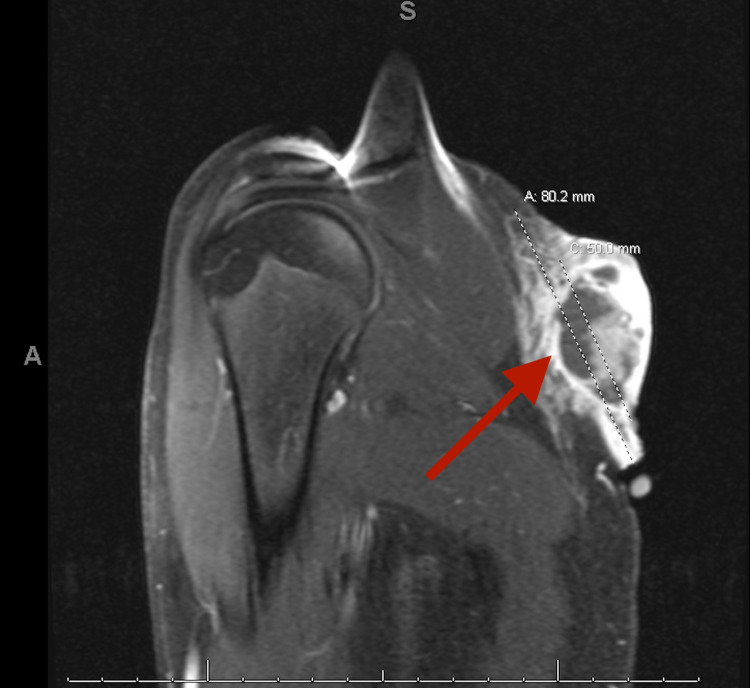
MRI of the mass Complex heterogeneously enhancing abnormality within the posterior upper left chest soft tissues with a multilobulated exophytic mass that protrudes posteriorly.
